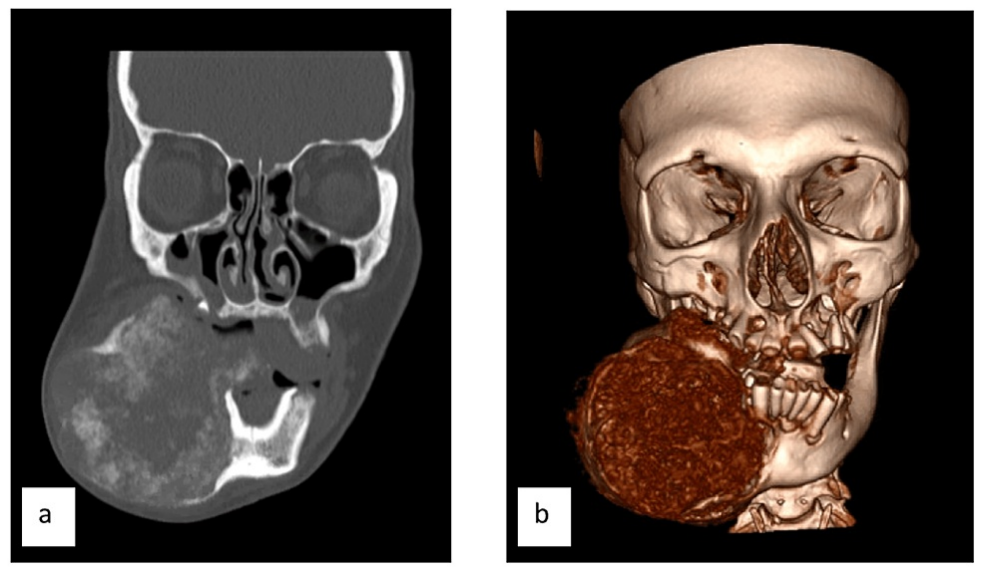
A. Depicts a coronal CT and B. shows a coronal reconstruction. Both images display thinning of the outer cortex of bone along the anterolateral margins of the mass with relative preservation of the cortex along its posterior margin.
